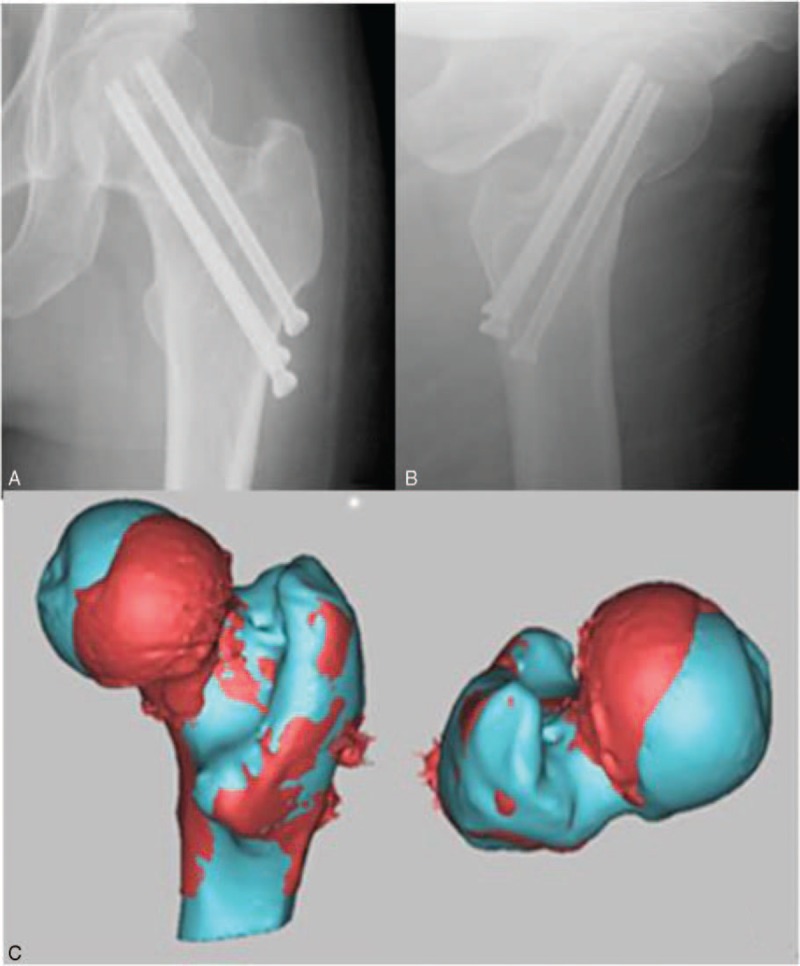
Antero-posterior and lateral postoperative radiograph of a left femoral neck fracture in a 55-year-old man (A and B), front and top views of proximal femoral 3-dimensional model and mirror model (C).
